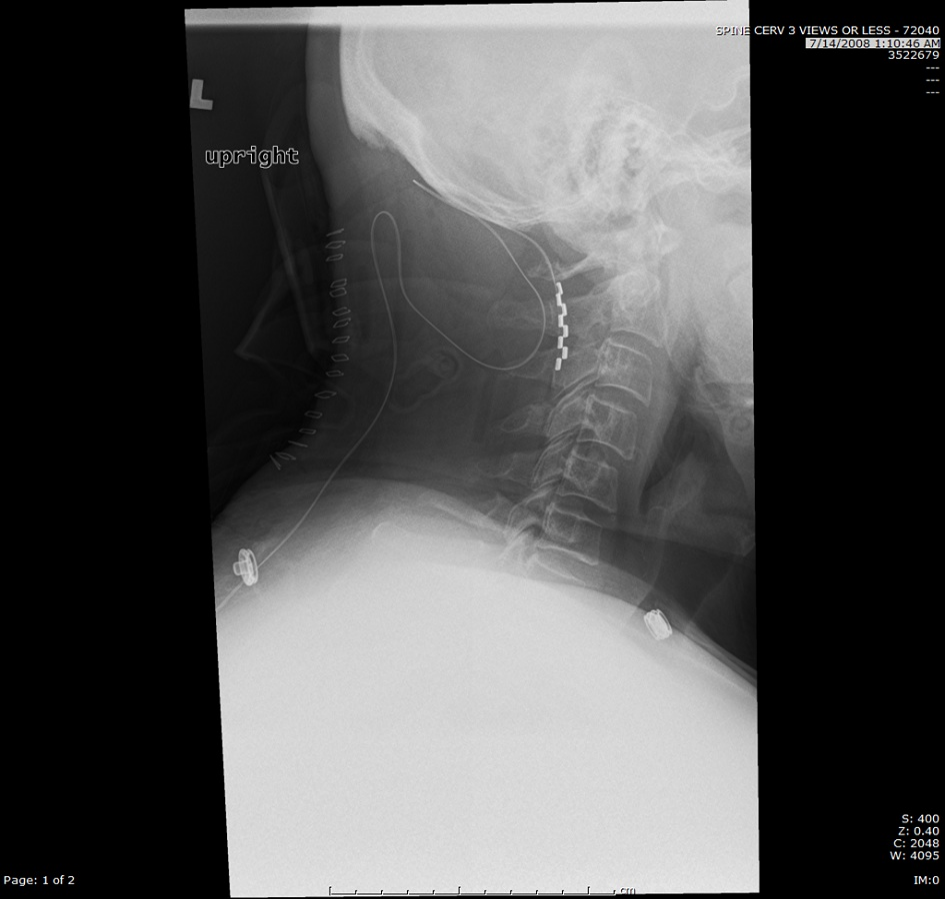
Lateral Cervical Spine X-Ray Dorsal column stimulator paddle lead at C1-C2
